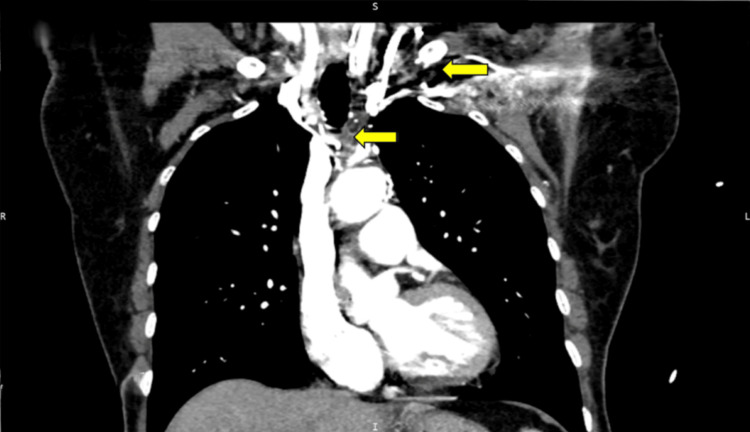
Coronal reconstruction of chest computed tomography angiography showing complete occlusion of the left subclavian and innominate veins Coronal reconstruction of chest computed tomography angiography in venous phase. The upper arrow indicates the expected anatomical location of the left subclavian vein, demonstrating complete absence of contrast opacification. The lower arrow indicates the expected anatomical location of the left brachiocephalic (innominate) venous trunk, similarly showing absence of opacification. These findings are compatible with complete venous occlusion secondary to iatrogenic dissection. A marked asymmetry is observed when compared with the normal opacification of the contralateral right-sided venous system, which serves as an internal anatomical reference. The superior vena cava remains patent, ensuring preserved central venous return through the right-sided pathway. Systematic pericardial assessment on this study documented the absence of significant pericardial fluid collection, with normal visualization of the pericardial layers and no focal pericardial thickening, loculated effusions, or localized hematoma, confirming the resolution of the mild pericardial effusion detected echocardiographically in the immediate post-procedure period
